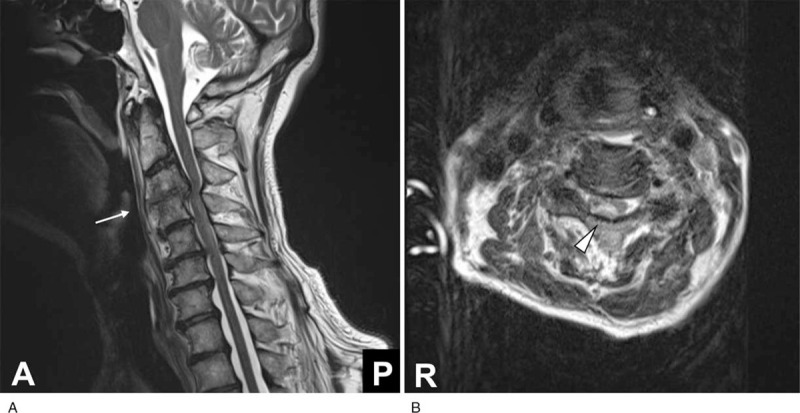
Magnetic resonance images of the cervical spine. In the T2-weighted sagittal image, vertebral osteomyelitis at the C3/4 level and compression of the spinal cord were detected (arrow) (A). The axial view of the slice shows myelomalacia at the compression site (arrowhead) (B).
